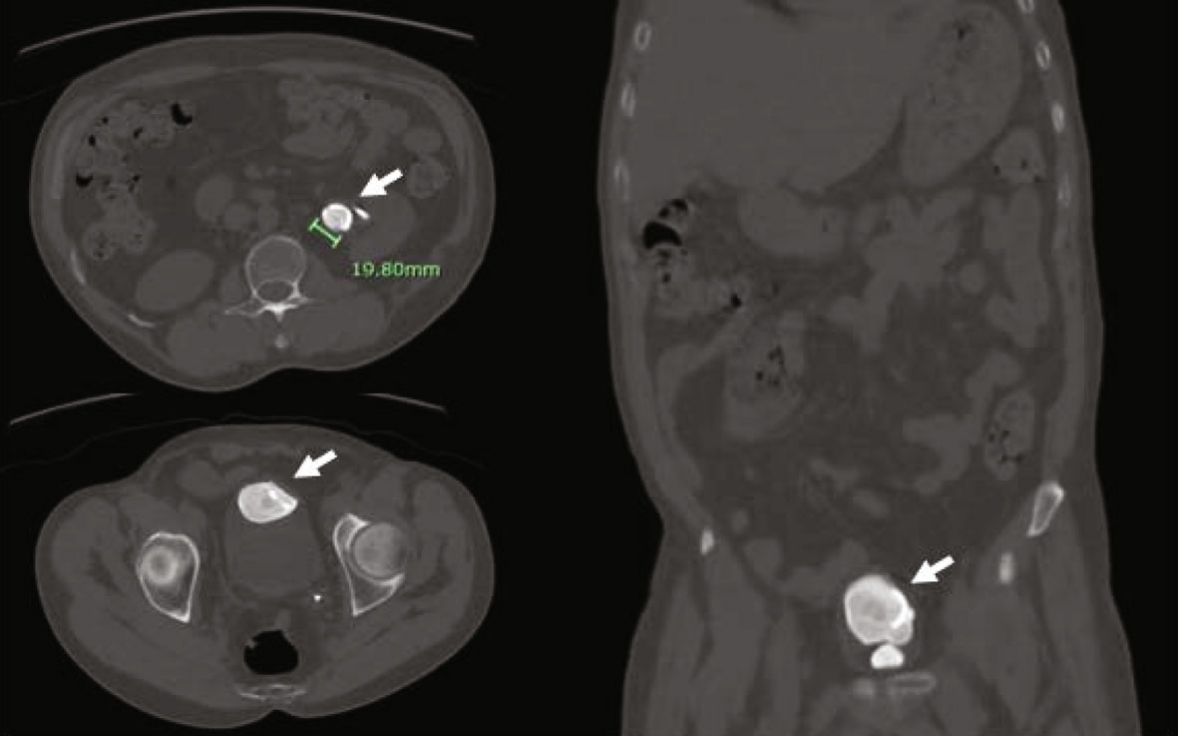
Patient 1 preoperative CT scan showing severe encrustation of the left ureteral stent at the proximal and distal coils (arrows).
